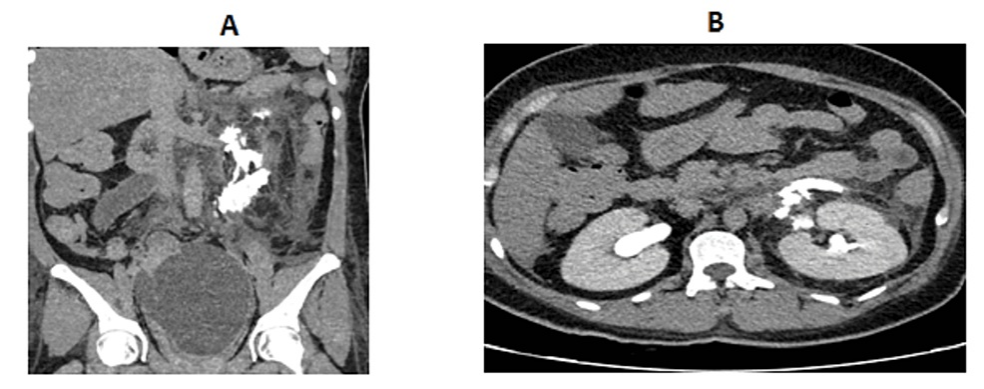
Delayed CT with IV contrast image showing contrast extravasation from the left renal calyceal system due to distal left ureteric obstruction secondary to pelvic collection (A) Coronal CT. (B) Axial CT. CT, computed tomography
